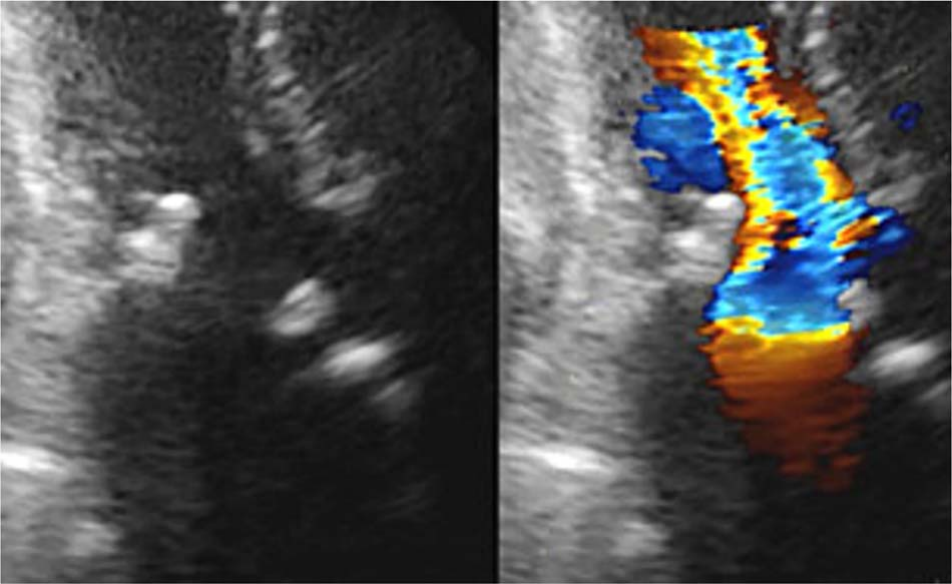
Postoperative transthoracic echocardiography: normal function of both prosthetic valves without dysfunction.
